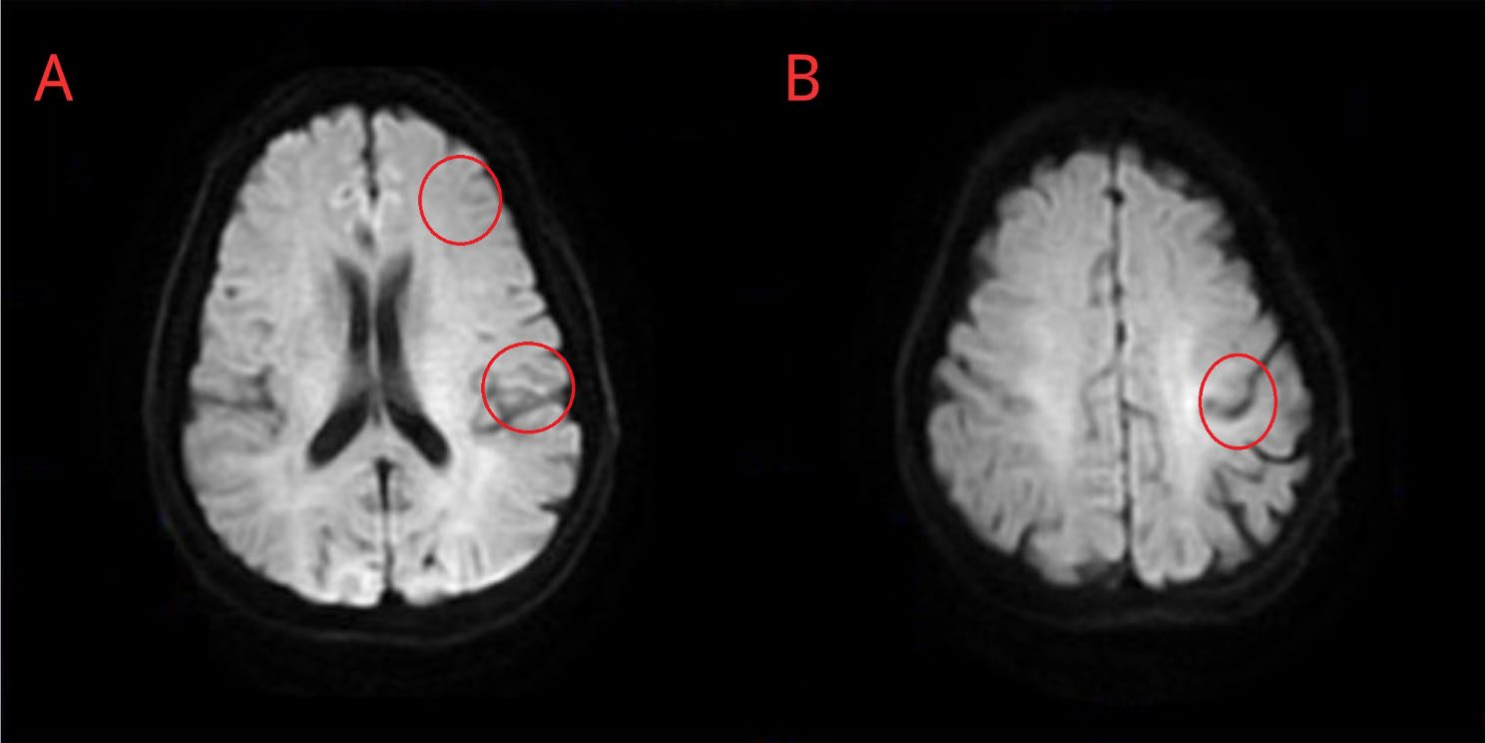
Brain MRI after AIS. The MRI demonstrates patchy signal intensity (red circles) in the left frontal lobe (A) and at the junction of the left frontoparietal lobes (B) on DWI, suggesting the presence of cerebral infarction at a very early stage
